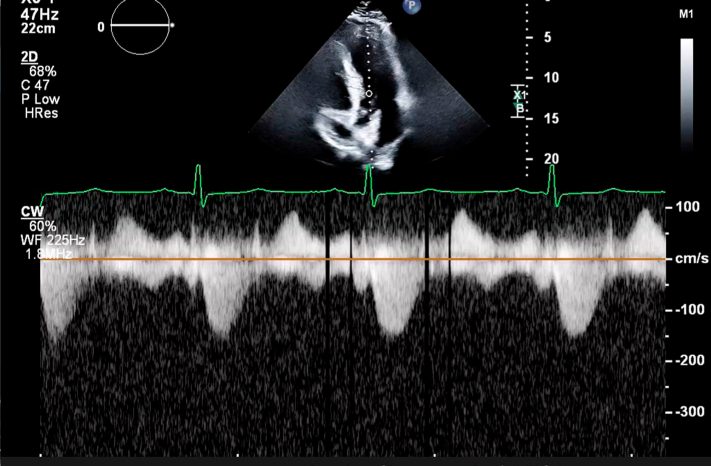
Athlete's Resting Left Ventricular Outflow Tract Gradient Continuous wave Doppler across the aortic valve displaying no significant left ventricular outflow tract gradient (<20 mm Hg).
